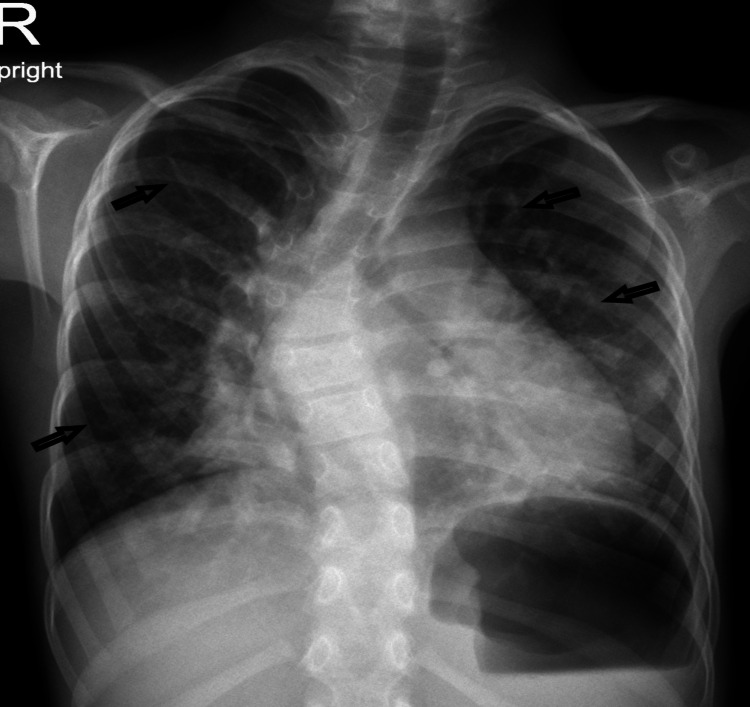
Chest X-ray showed inhomogeneous air shadowing in both lungs and marked mid-thoracic rightward scoliosis
